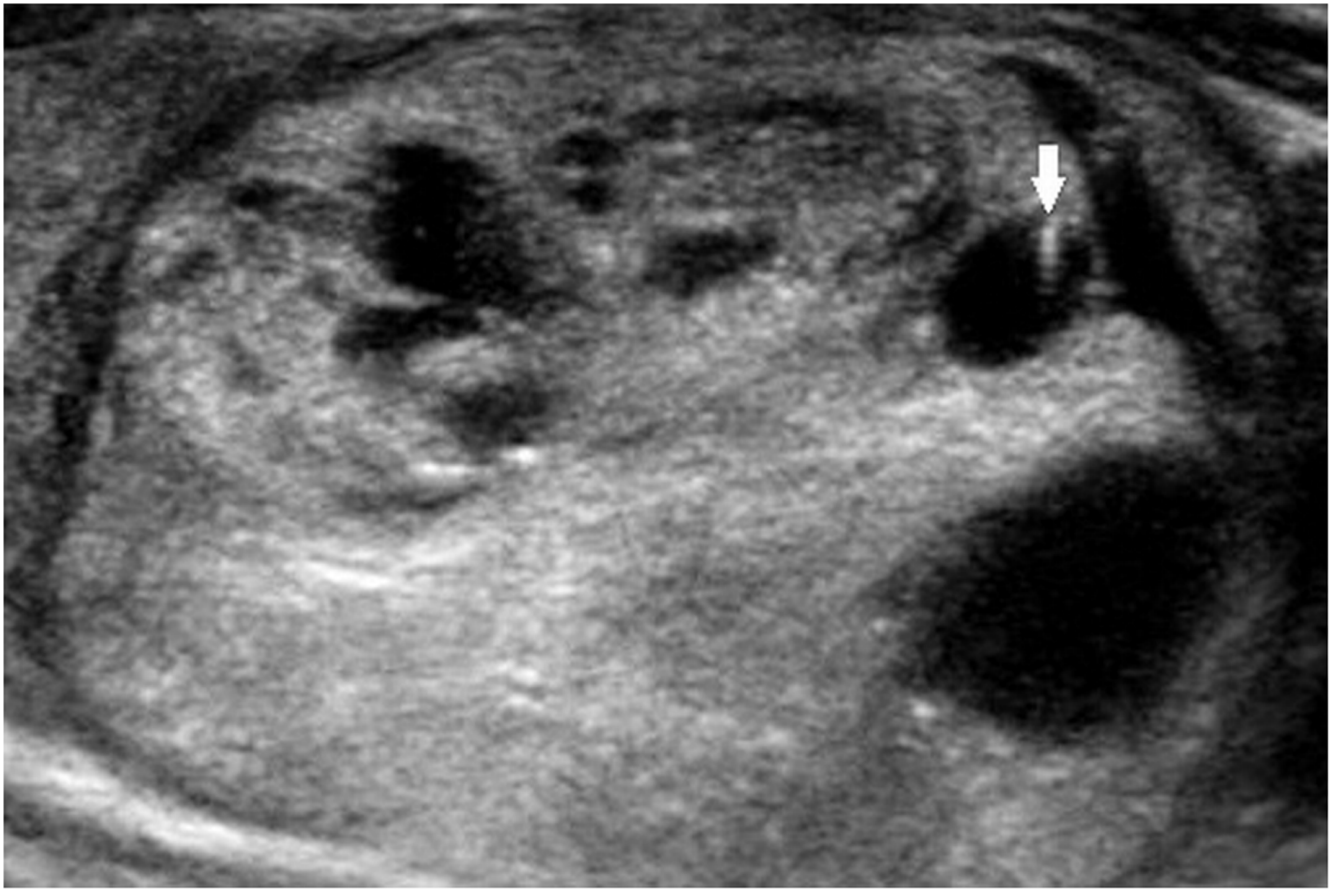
A 41-year-old man with multinodular goiter. Longitudinal sonogram shows an 36-mm, smooth, mixed isoechoic nodule in the right thyroid lobe. Echogenic focus is located at the margin of solid component (solid arrow); comet-tail artifact is located within the cystic component (Type 2).
